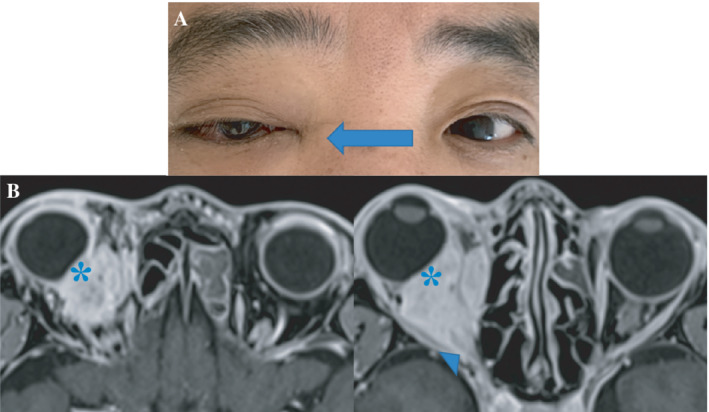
(A) Right eye ptosis and right eye abduction limitation. (B) The orbital magnetic resonance image showed mass in the right orbit excluded the right eye (asterisk) and extended to the apex of the orbit (arrowhead)
